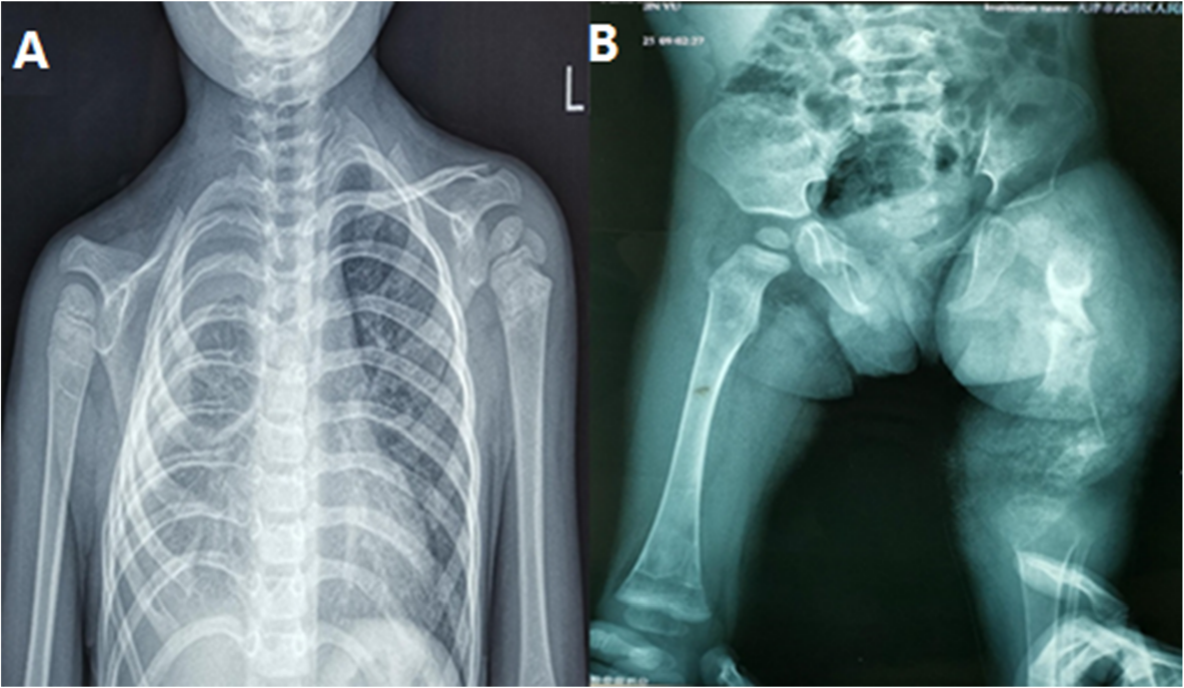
(a) X-ray showing osteolysis in the right clavicle. A large pleural effusion was observed on the right-side, and the left lung was significantly compressed. (b) X-ray showing the fracture of the left femur
